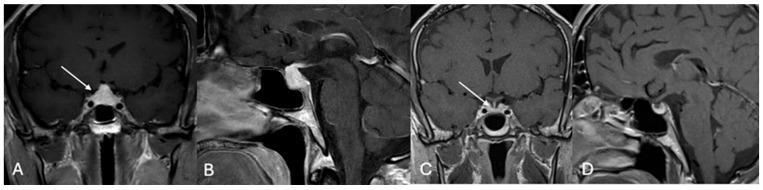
Hypophysitis. In this case of hypophysitis of a 40-year-old male patient, surgery was avoided due to an accurate endocrinological diagnosis. The patient received high-dose corticosteroid therapy, leading to complete disease resolution. (A,B) Coronal and sagittal MRIs show an enlarged contrast-enhancing pituitary gland and prominent enhancing pituitary stalk; these findings initially made it suspicious to diagnose a PitNET. (C,D) MRI follow-up after 15 months showed regression, and hormone replacement therapy was not required. This highlights the importance of precise diagnosis and close collaboration between endocrinologists, radiologists, and neurosurgeons in managing such cases without surgical intervention.
